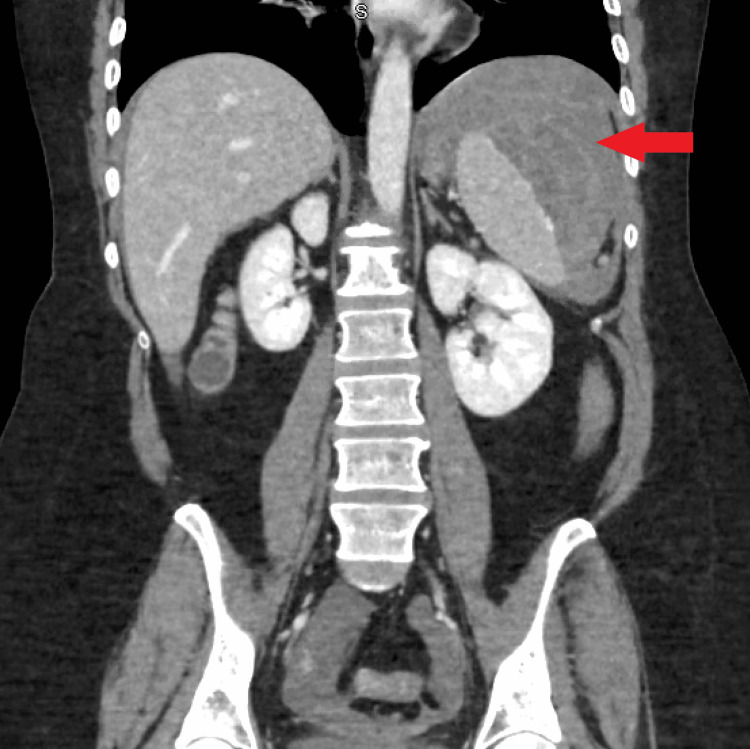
Coronal CT abdomen with contrast showing subcapsular perisplenic hematoma (red arrow).
